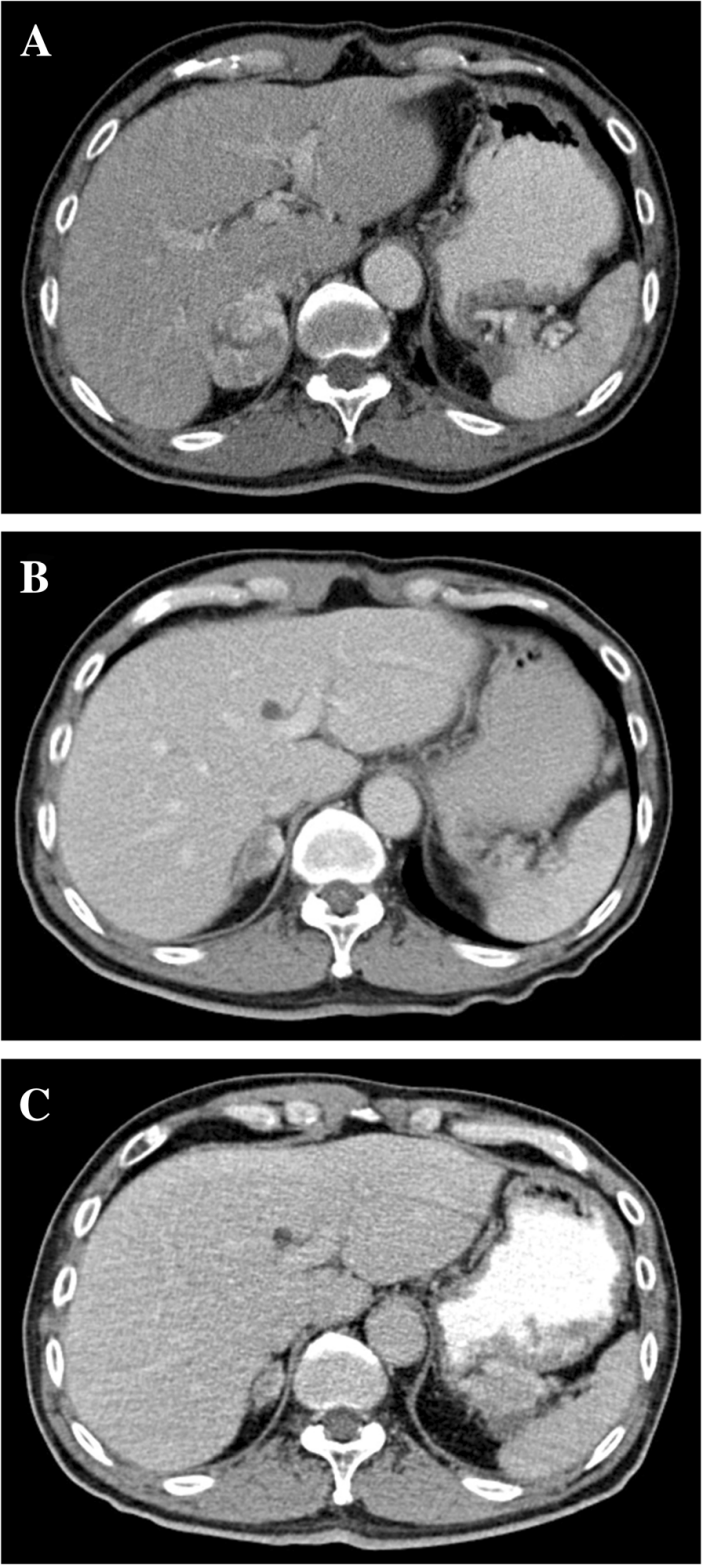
Abdominal CT examination revealed spontaneous regression of adrenal metastasis (left to right). a. Adrenal metastasis measuring 5.7 cm in diameter. b. Adrenal metastasis measuring 3.4 cm in diameter. c. Adrenal metastasis measuring 1.8 cm in diameter
